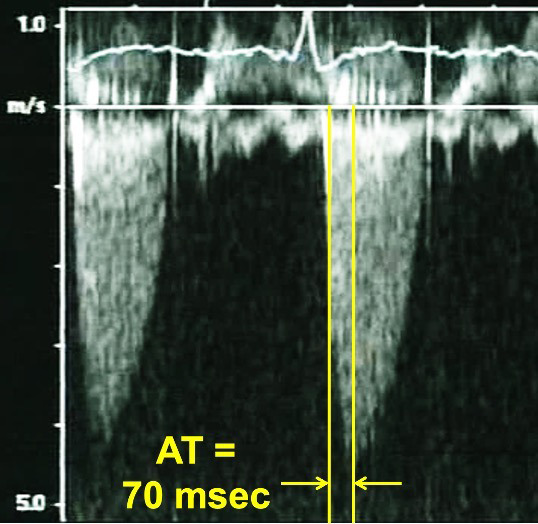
Continuous Wave Doppler of a normally functioning aortic
